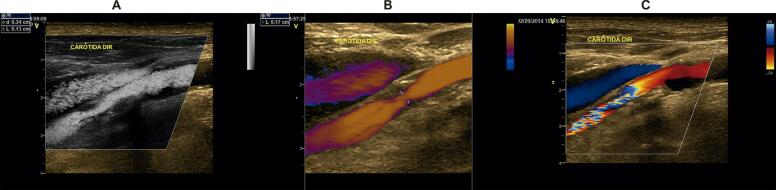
Medida da luz residual (A) com B-*flow*, (B) B-*flow *angio e (C) Doppler colorido. Nas técnicas relacionadas com o B-*flow*, há menor sobreposição da cor sobre a placa, sendo o método preferível para a medida da luz residual.
